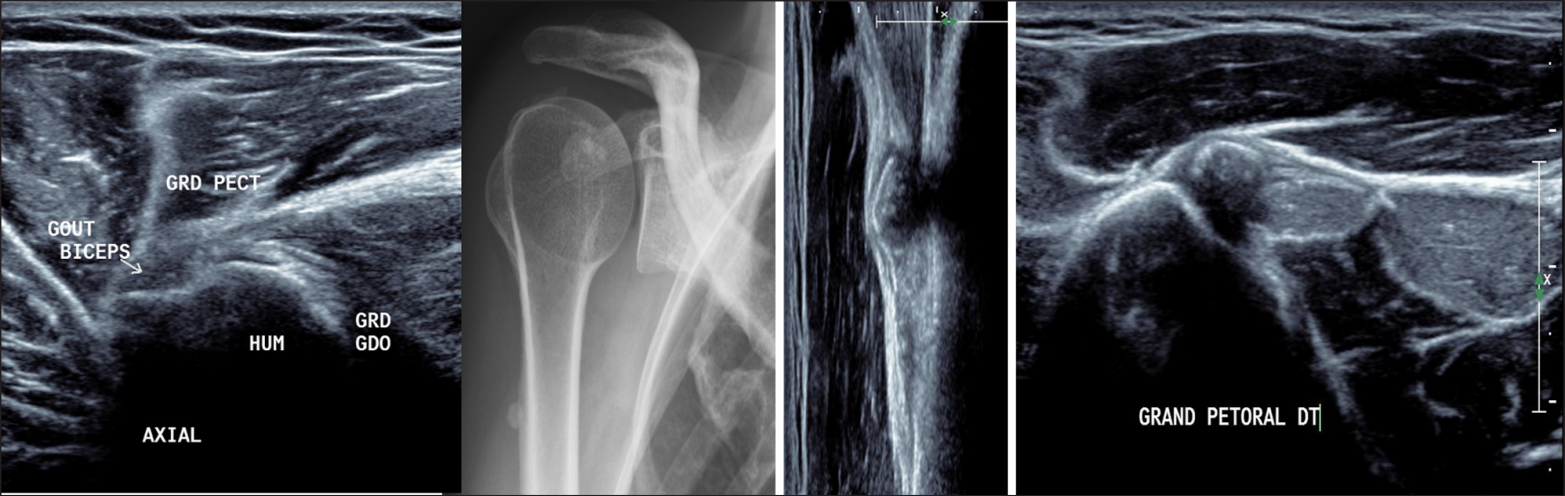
Axial view of the normal anterior part of the humerus in external position (a) with hydroxyapatite deposits in X-rays (b) and US in sagittal and axial view (d). GRD/GDO: Teres Major/Latissimus Dorsi, GRD PECT: Pectoralis Major, HUM: Humerus, Court Biceps: Short head of the biceps.
